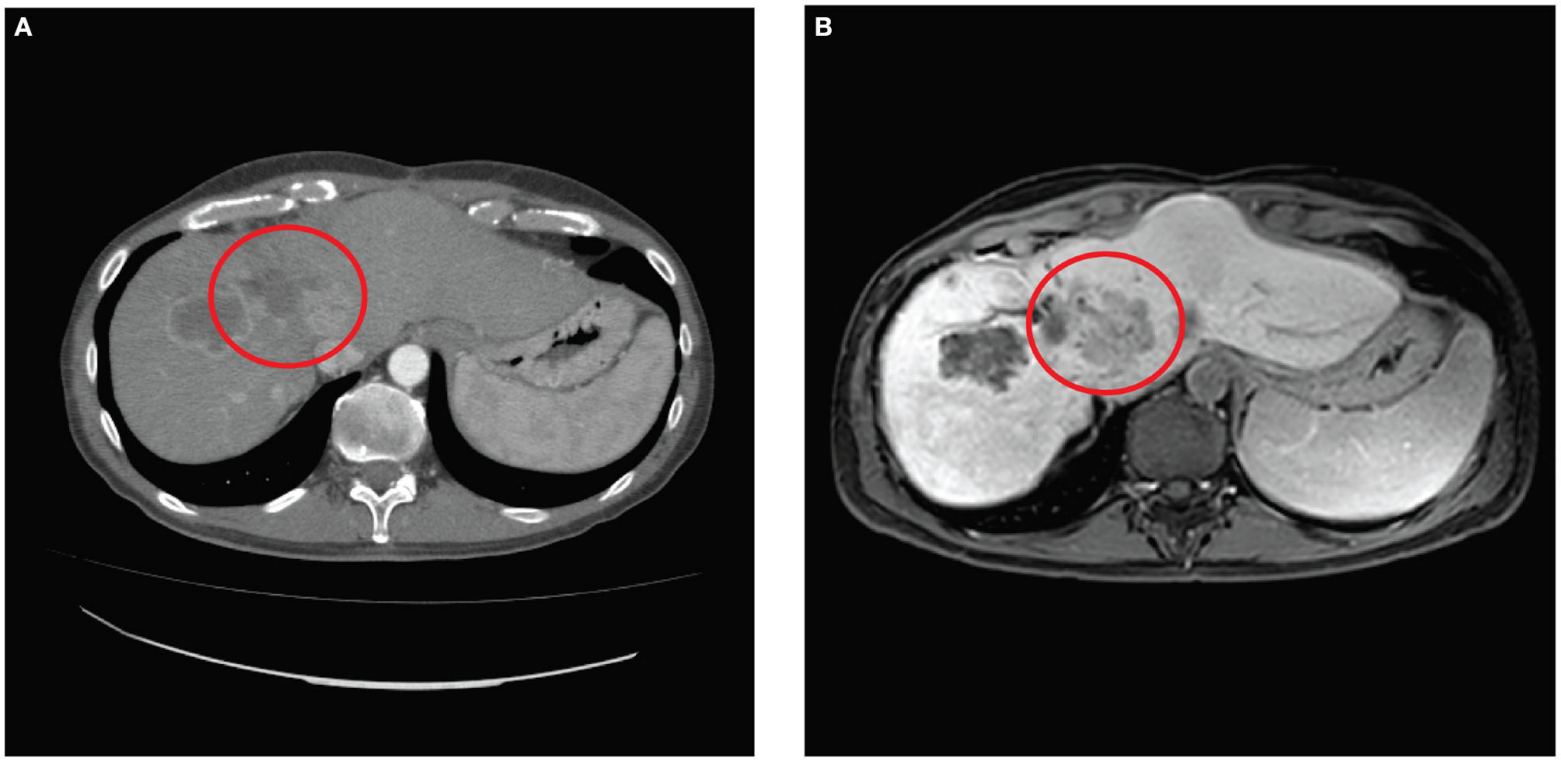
(A) Contrast computed tomography scan performed with contrast 6 months after surgery and (B) magnetic resonance scan performed with gadolinium contrast T1 WI 10 months after surgery show in both that the electrochemotherapy-treated neoplasia resulted permanently ipodense indicating the necrosis of the lesion.
